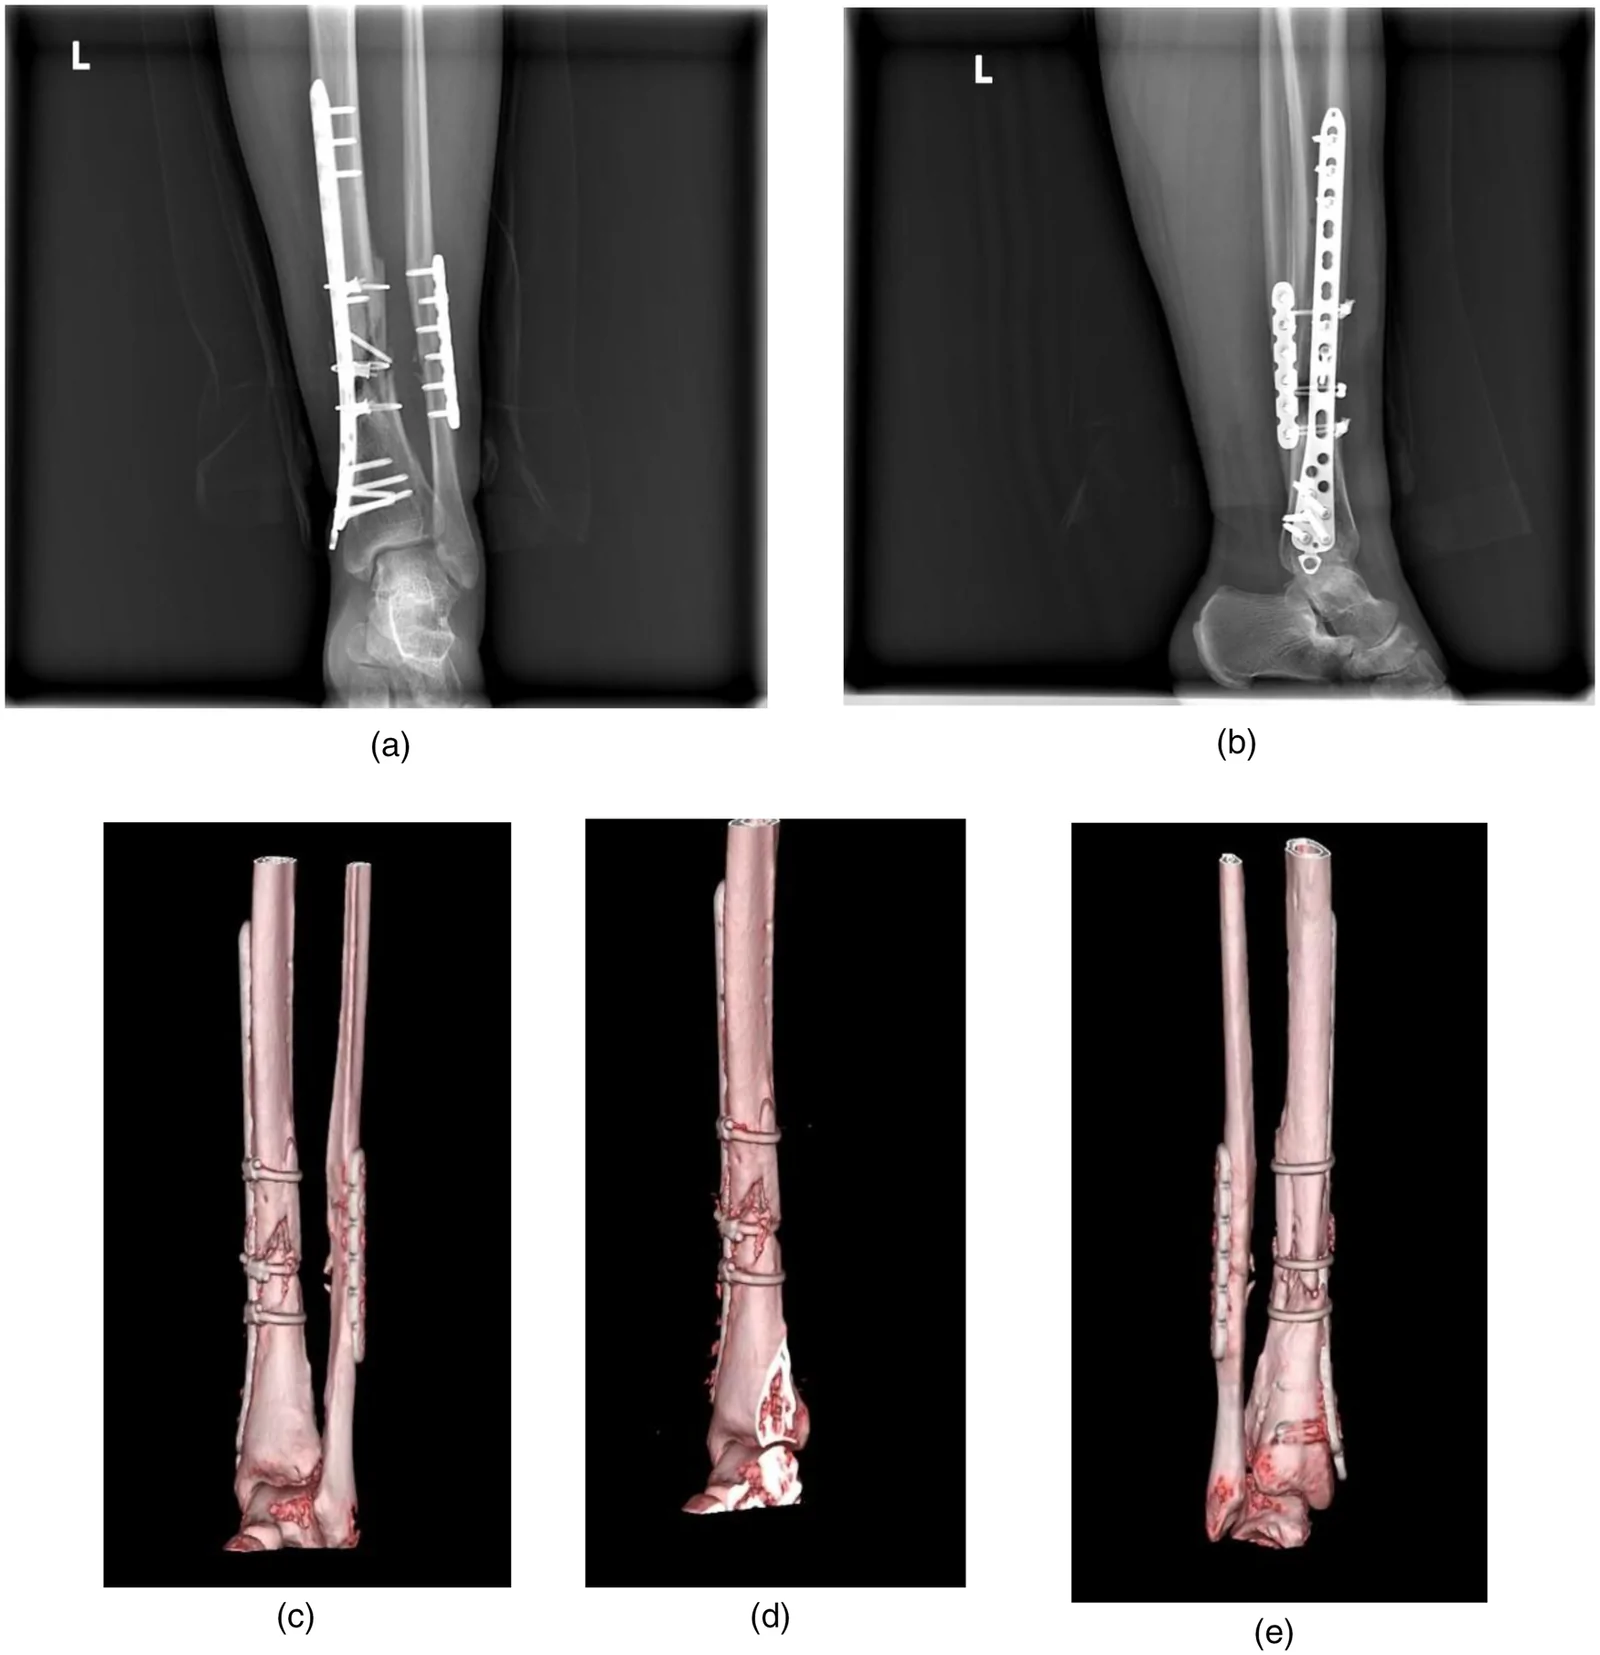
(a,b) on x-ray and (c–e) on CT, fracture healing at the tibial fracture site remains indistinct, while laboratory tests in Table 1 indicate no evidence of infection.
